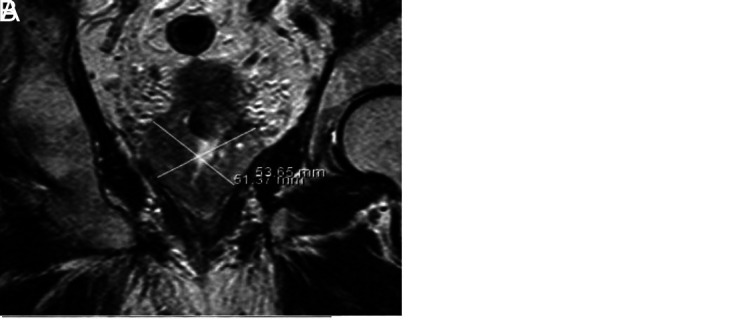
(a) Axial T1-weighted MR images show pre-procedure. (b) Coronal T1-weighted MR images show post-procedure.
